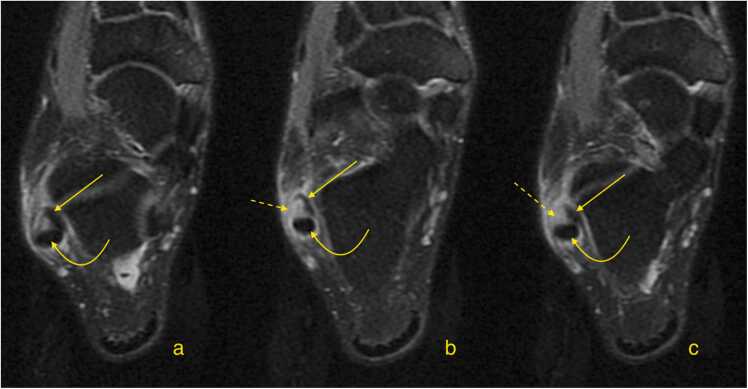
A patient with chronic lateral ankle pain. Magnetic resonance imaging (a-c proton density weighted with fat suppression) revealed a peroneus brevis split tear just below the level of the lateral malleolus (straight arrows) with *boomerang sign*, accompanied by tenosynovitis (dashed arrow). The peroneus longus tendon (curved straight arrow) appears normal.
